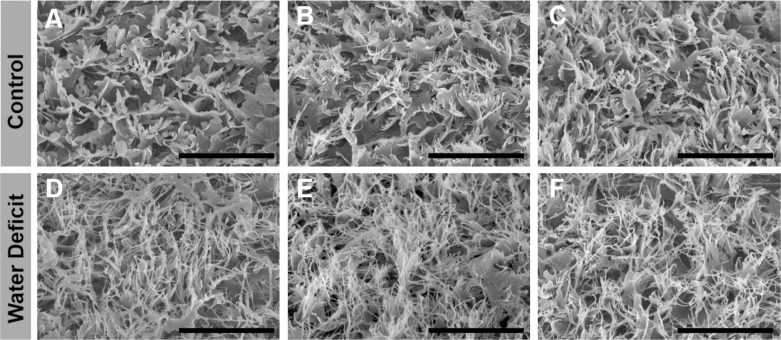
Ultrastructural morphology of cuticular waxes on grape berries at 113 days after anthesis (DAA). SEM images of the cuticular wax ultrastructure on grape berries of grapevines (*Vitis vinifera* L.) exposed to two irrigation treatments: (A–C) well-irrigated (control, CT); (D and E) deficit irrigated (water deficit, WD). Each image represents one biological replicate. The scale bar represents 5 µm in all images.
